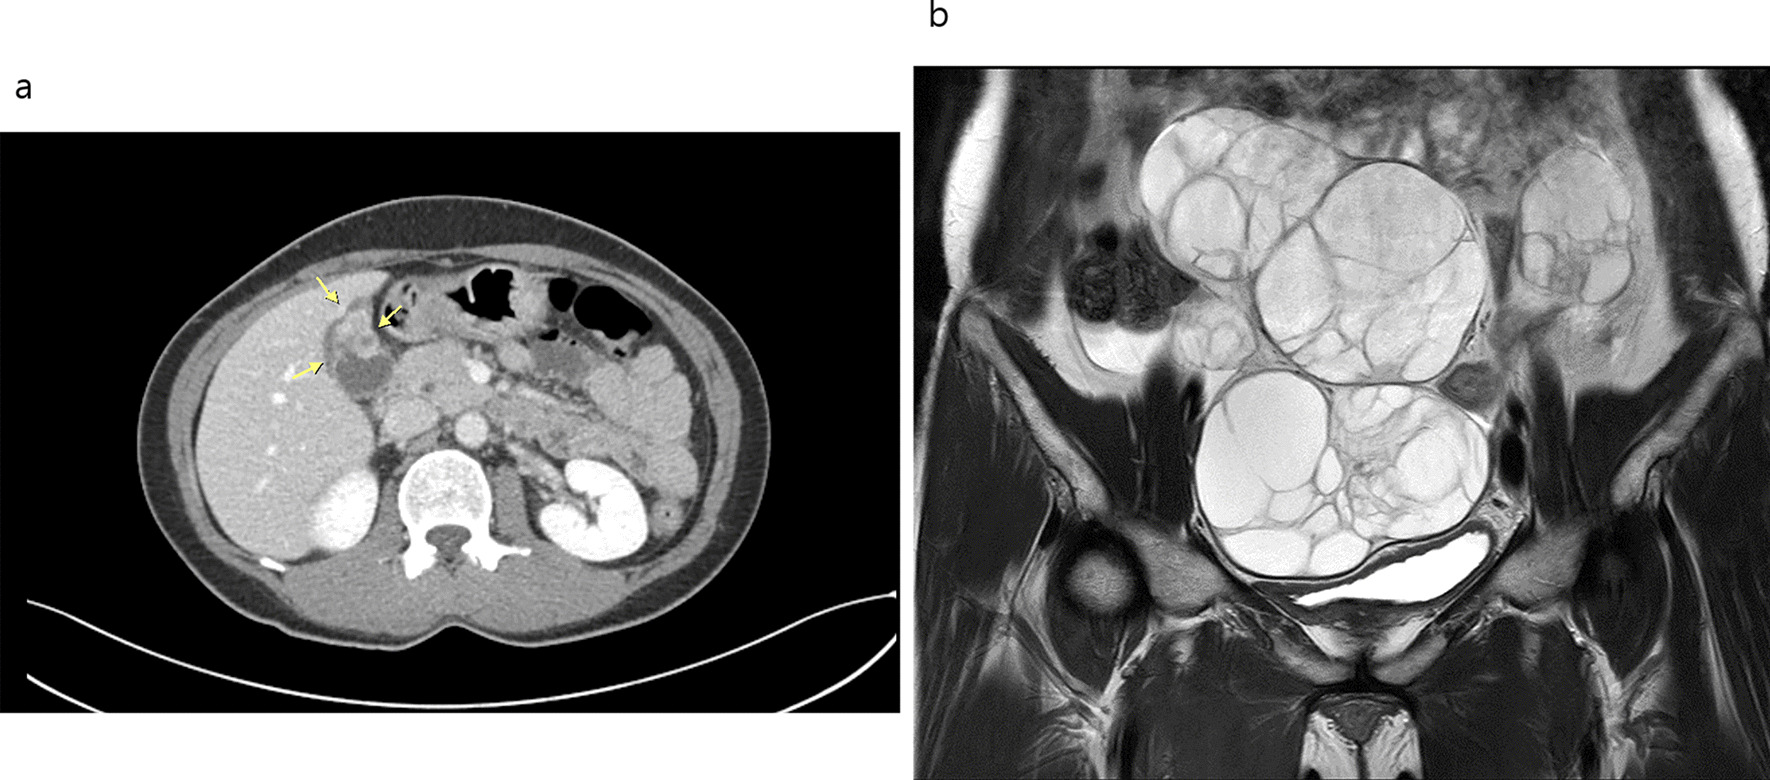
Magnetic resonance imaging findings of large bilateral ovarian tumors (a) and abdomen and pelvis computed tomography scan with intravenous contrast demonstrating wall thickening of the gallbladder (b) (arrows indicates wall-thickened gallbladder)
